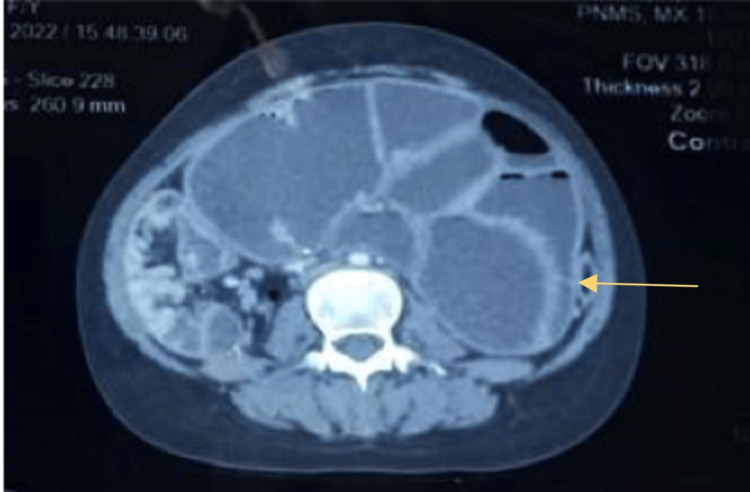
CECT abdomen - axial section CECT - contrast-enhanced computed tomography
